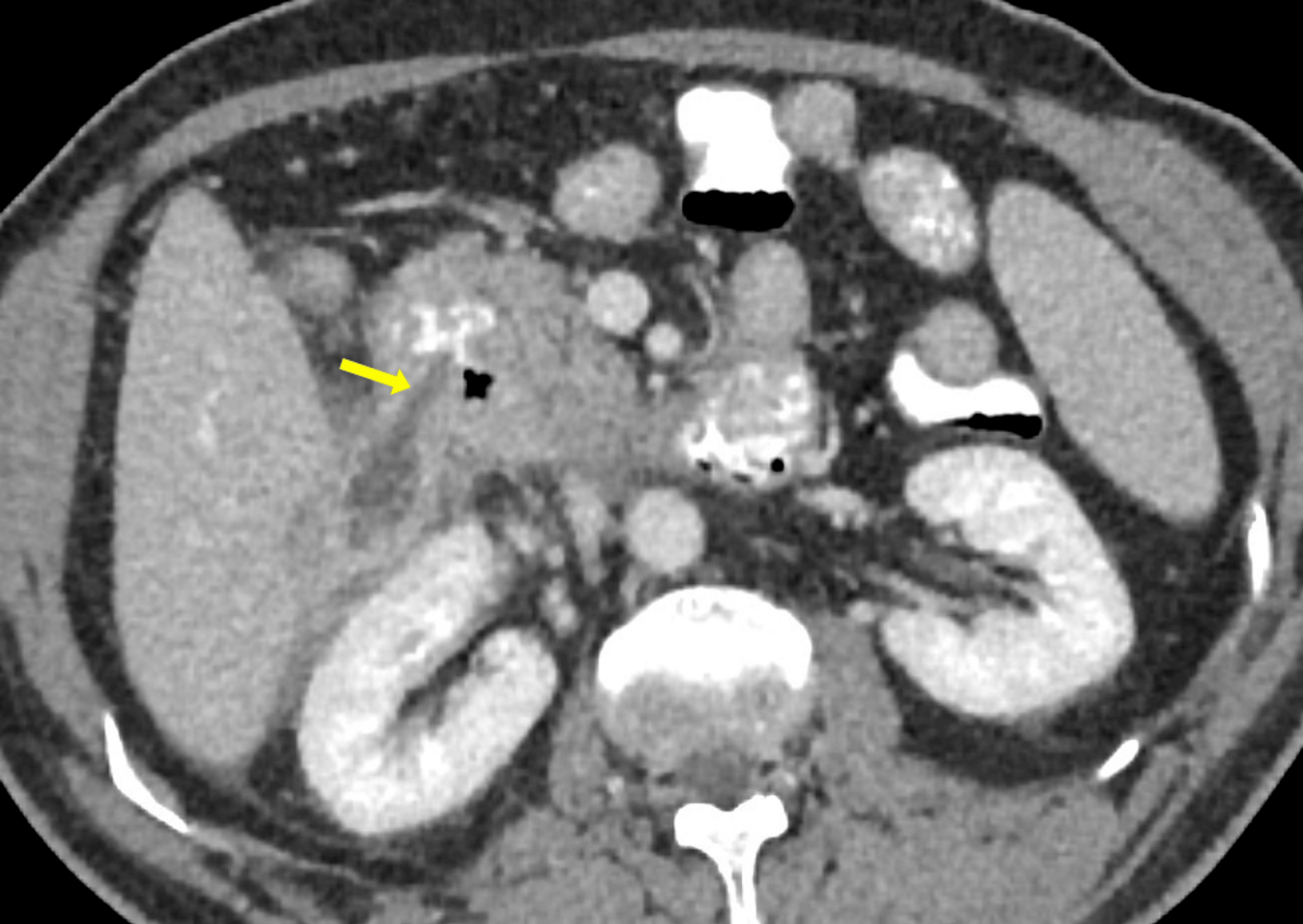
Contrast-enhanced CT scan with intravenous and oral contrast (axial section) showing a narrow tract (yellow arrow) communicating between the lateral wall of the second part of the duodenum and the diverticulum.
